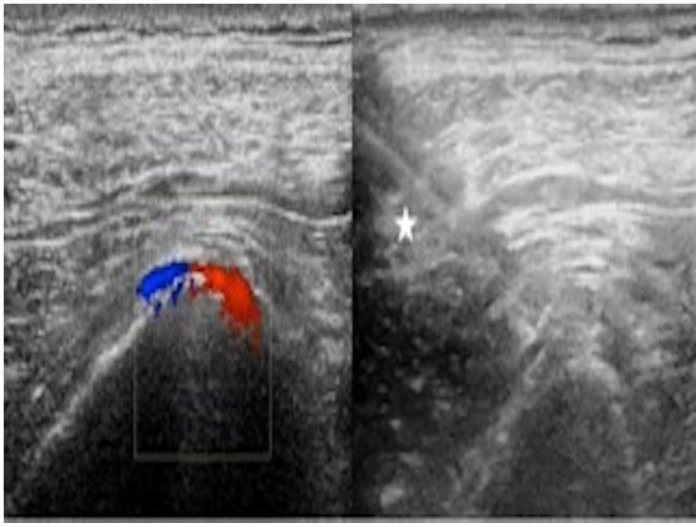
Color Doppler and gray-scale ultrasound of the gluteal region demonstrating patent superior gluteal artery that was accessed under ultrasound guidance (*).
